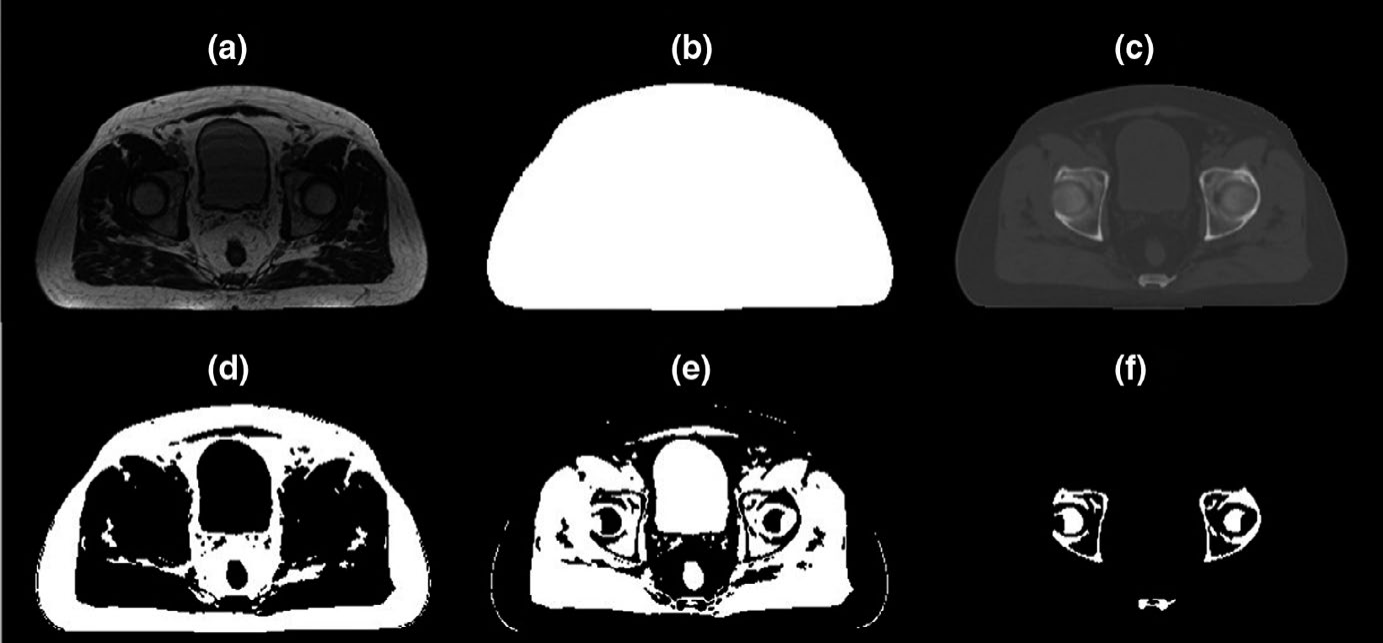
Classification maps generated for a coregistered MRI‐CT atlas. (a) original TRUFI MRI, (b) body contour, obtained using ViewRay treatment planning system, (c) deformed planning CT, (d) fat, (e) muscle, and (f) bone classification maps
